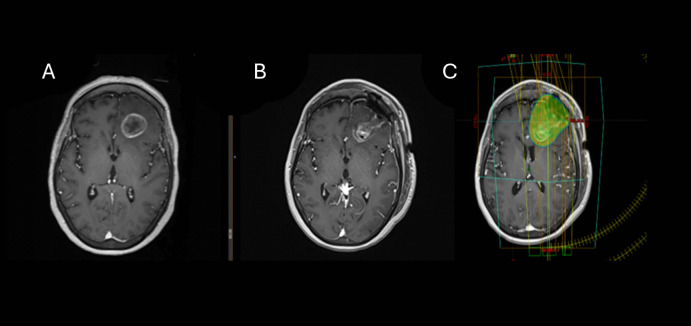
Brain MRI of a 46-year-old patient with luminal B breast cancer. Presenting symptoms of brain metastasis were headache and confusion (A). She underwent craniotomy for resection of a single brain metastasis at the left frontal lobe (A, B). The resection cavity was treated to a total dose of 30 Gy in 5 fractions, including the corridor (C). No evidence of local failure at time of last follow up
